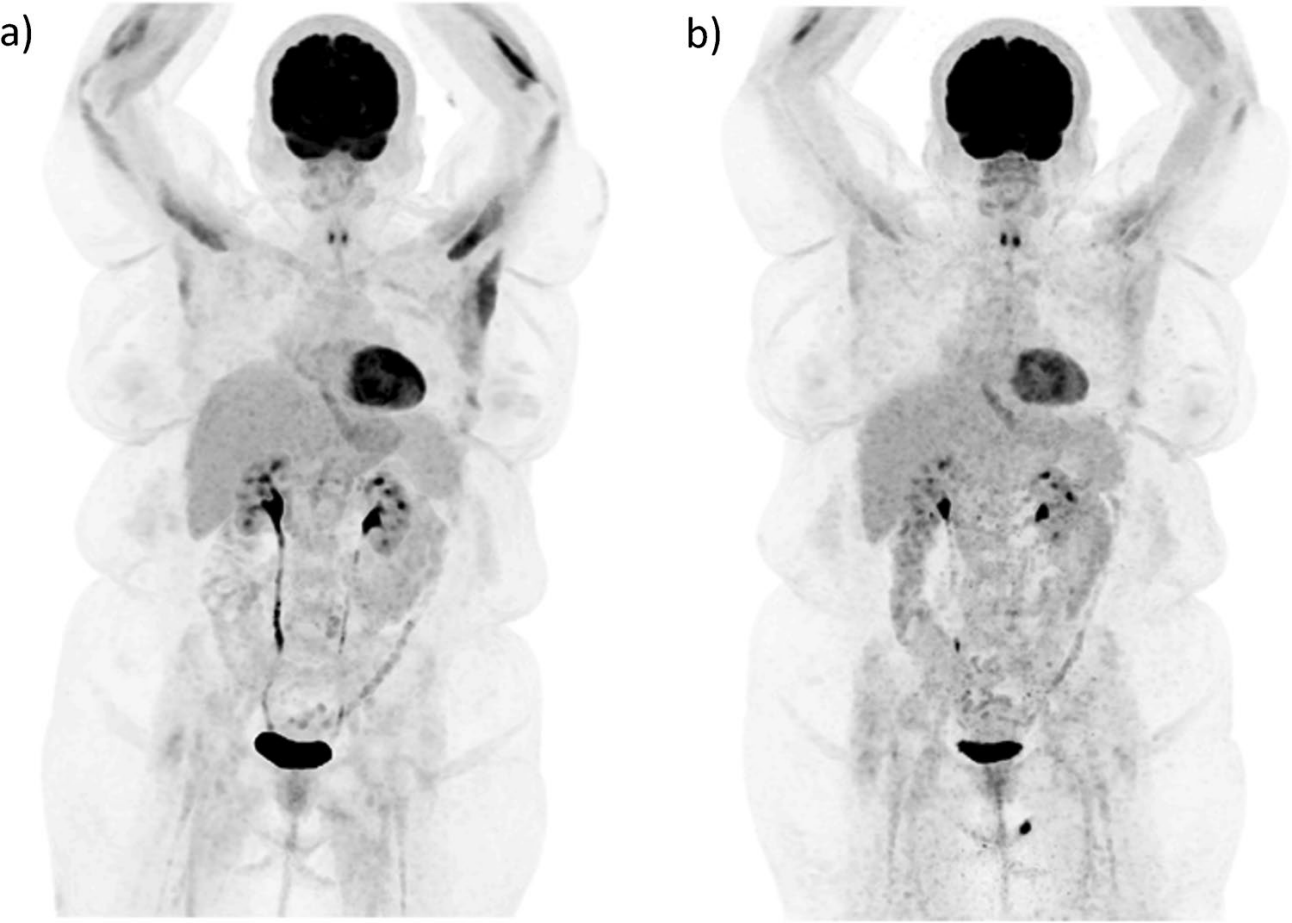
Example ^18^F-FDG patient (~ 2.6 MBq/kg and 10-min total scan time in both systems) acquired on the 6-ring Gen2 DMI (a) and on the 5-ring configuration (b) of the same system, reconstructed with QClear (beta 450) and data-driven respiratory motion correction (MotionFree, GE Healthcare)
